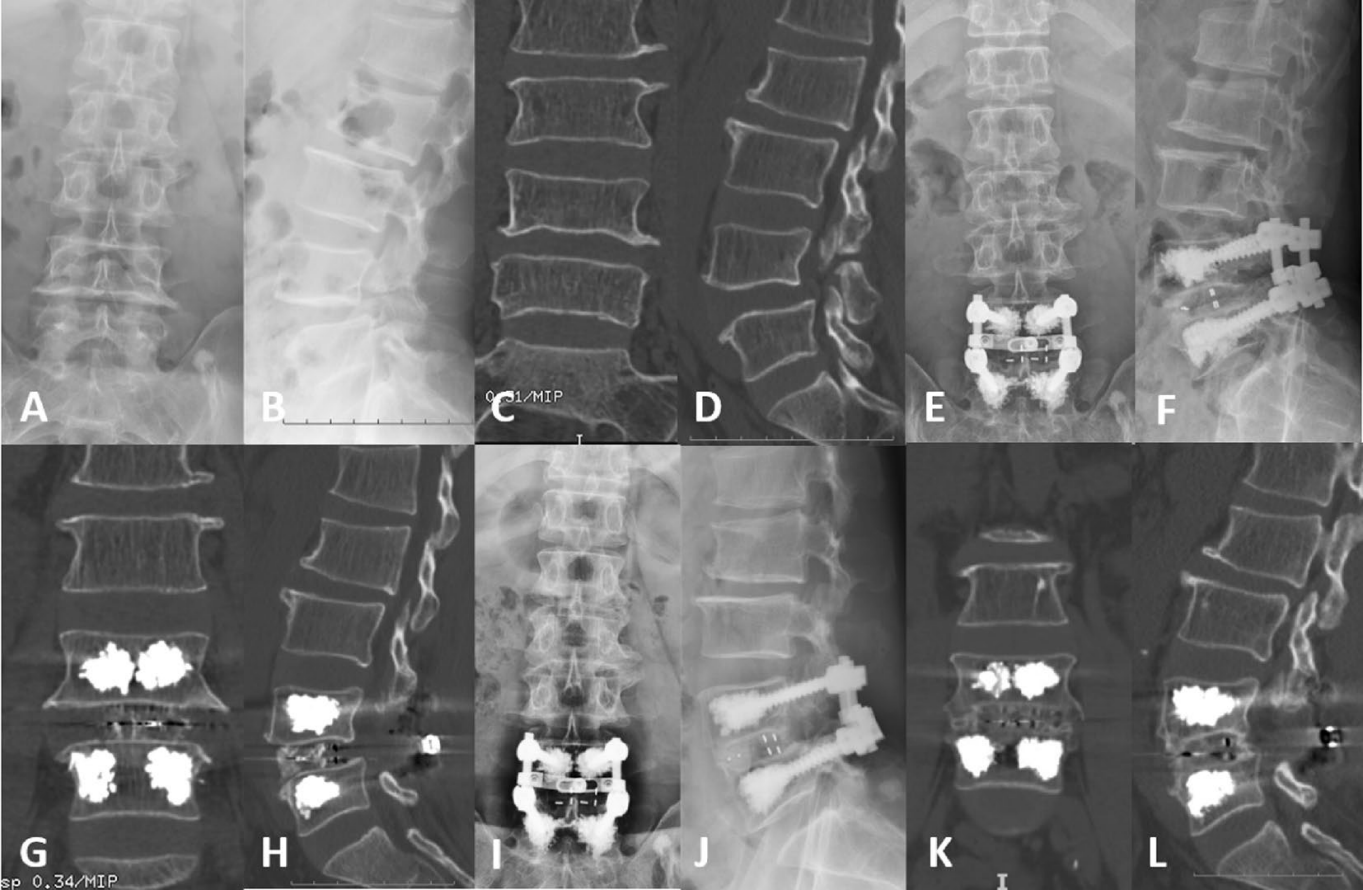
Radiological images of a representative case with PMMA-PS. (A–L) A 62-year-old osteoporotic male with isthmic spondylolisthesis in the L4/L5 segments. (A–D) Preoperative X-rays and CT showed forward slip of the L4 vertebra and L4 spondylolysis. (E–H) Postoperative X-ray and CT showed that the internal fixation position was good and that the bone cement was in the vertebrae. (I–L) Postoperative X-ray and CT at 36 months after fusion surgery showed that the internal fixation device did not loosen or break, the vertebra did not show slippage, the intervertebral fusion was good, the bone cement was well filled, and there was no obvious cement leakage.
